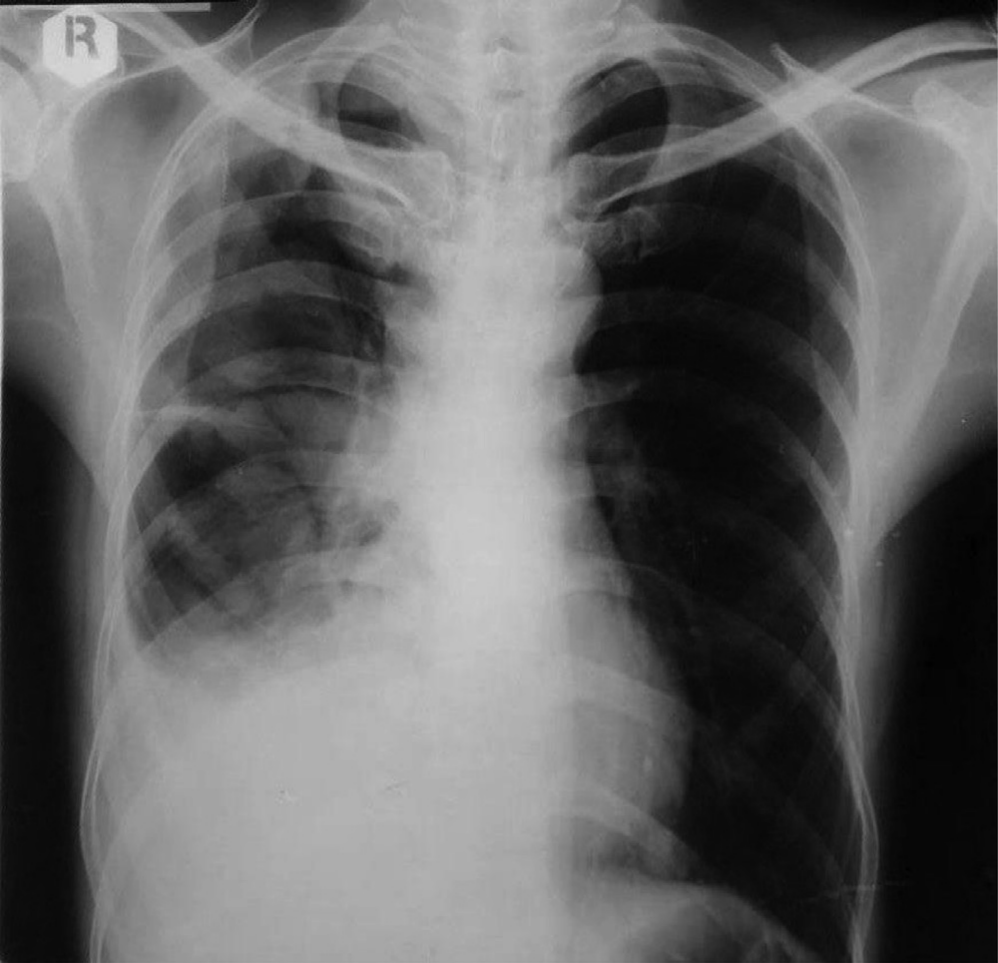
Pleural effusion, fissure thickening, and inhomogeneous opacities on the right lung were seen in the chest X-ray. A nodule was visible in the paracardial site on the left. Left lung hyperaeration was also evident.
